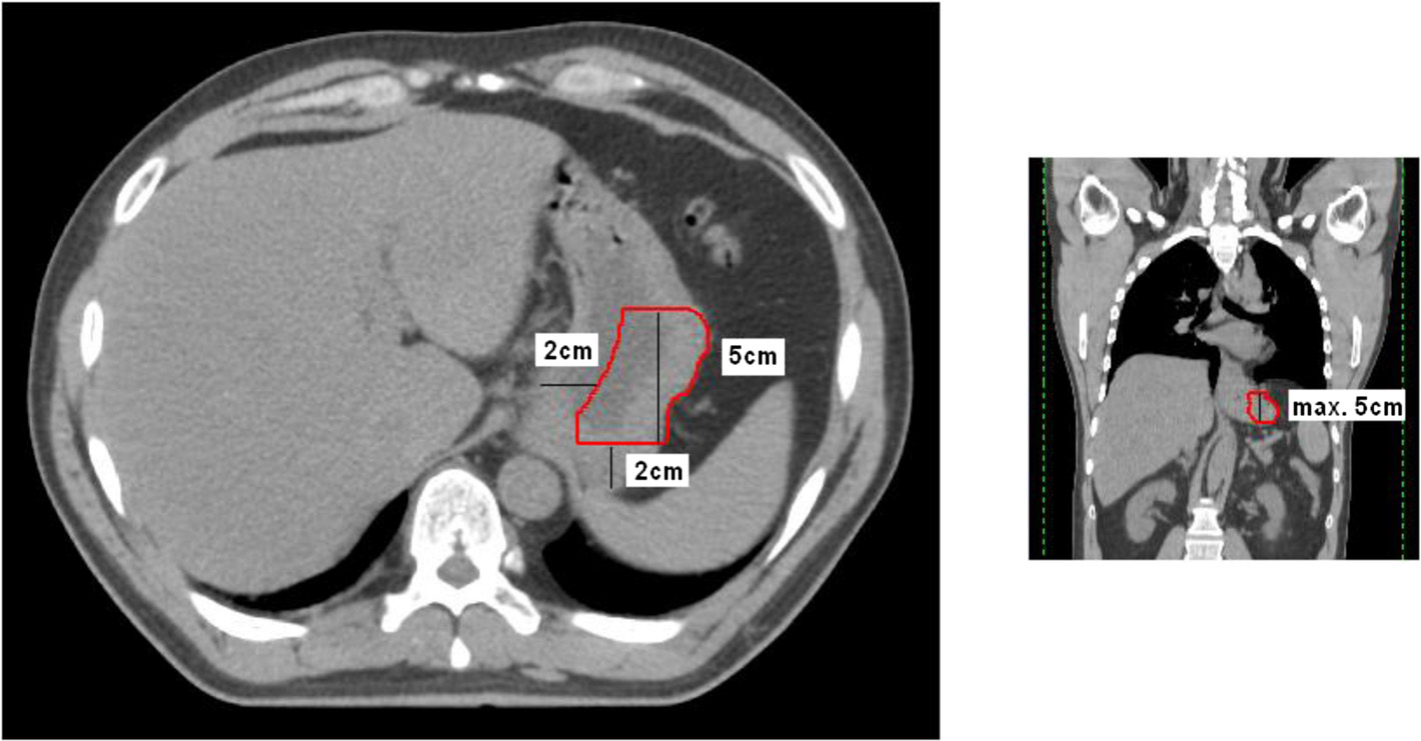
Example of a planning CT in which the future anastomotic region is drawn.
